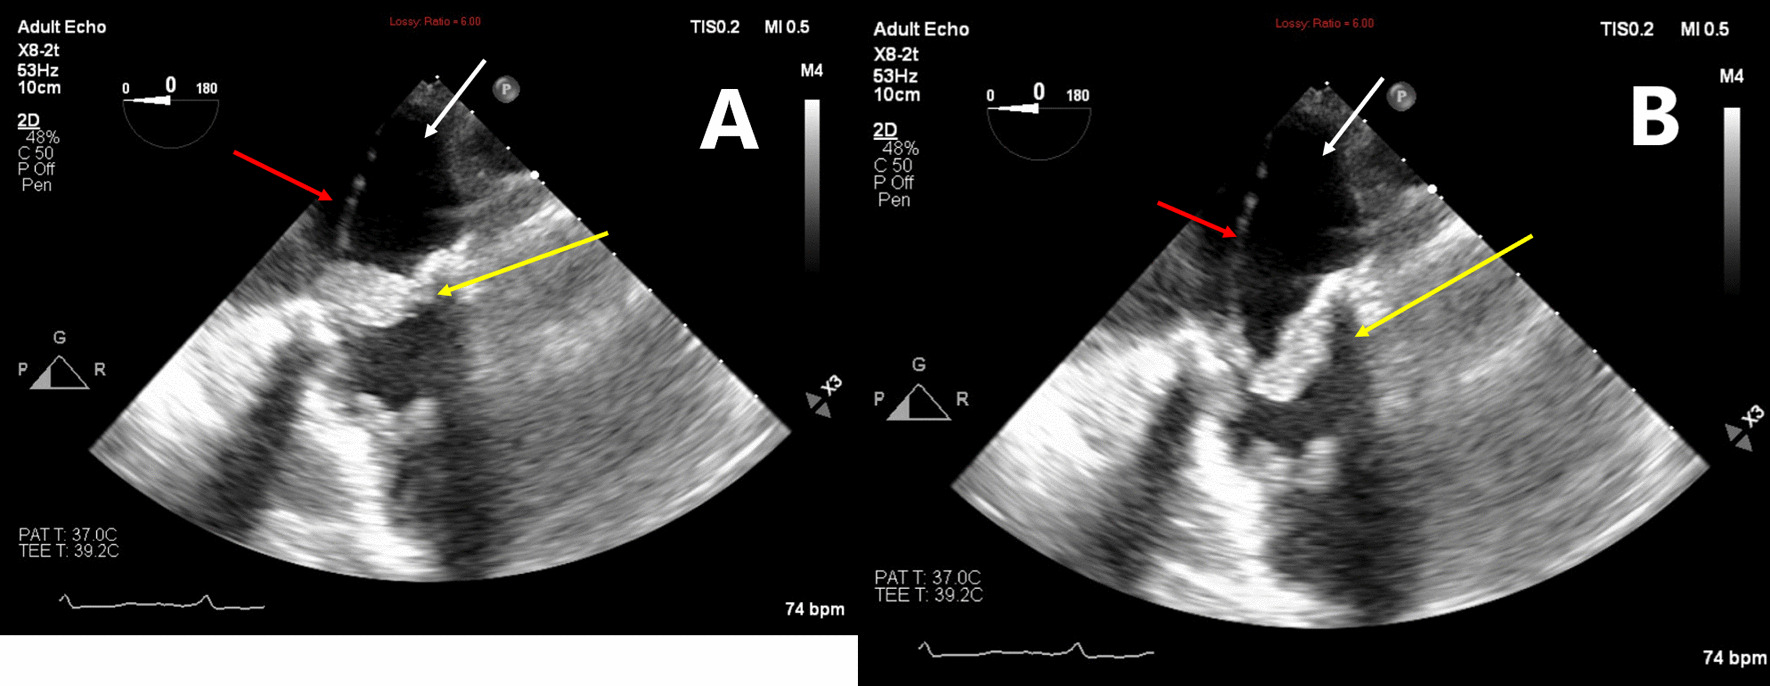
Transesophageal echocardiogram images during systole (a) and diastole (b). Large vegetation is attached to the pacemaker electrode (red arrow) and the prosthetic tricuspid valve (yellow arrow), resulting in severe tricuspid stenosis. The white arrow points to the right atrium
